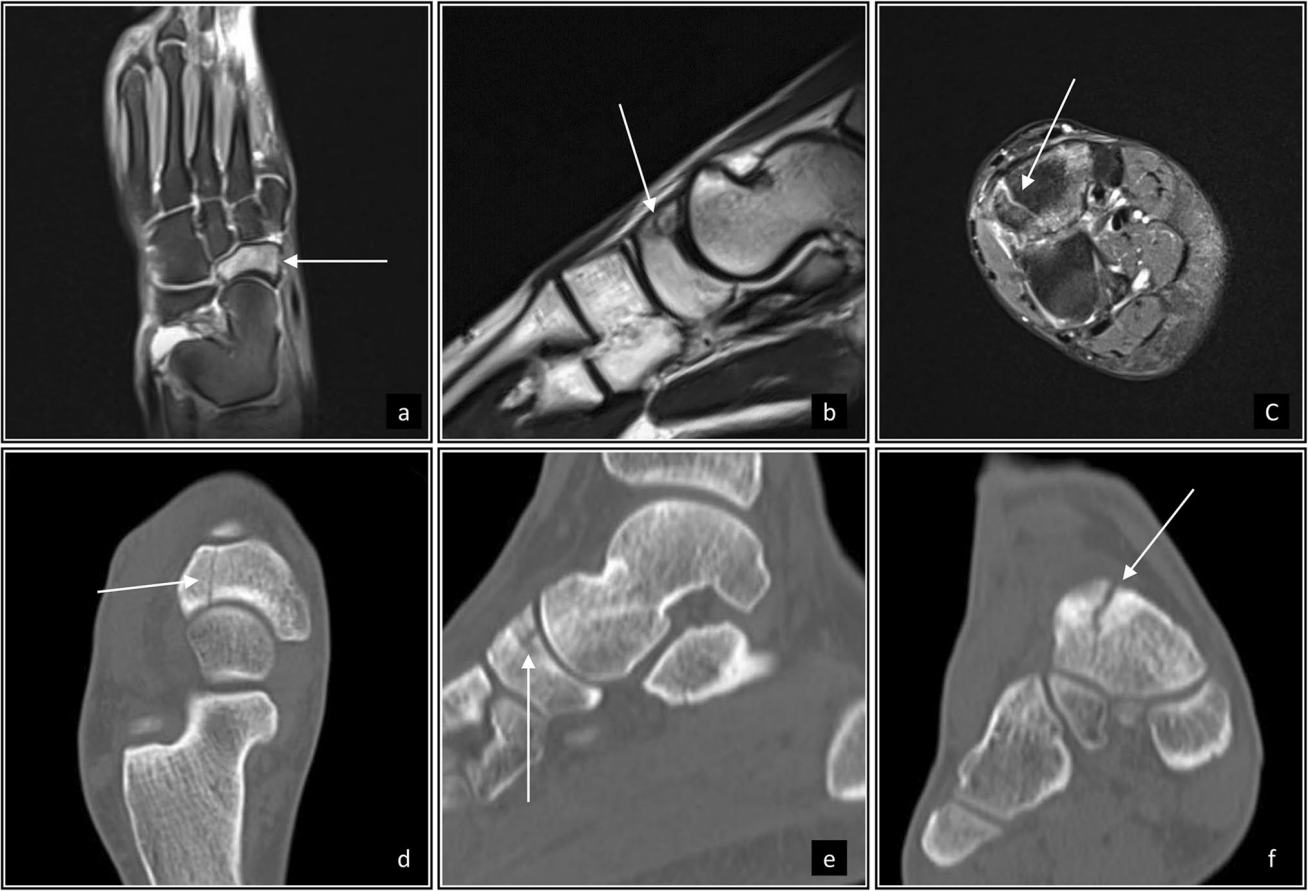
Images from Case 1. Sagittal (a), coronal (b), and long axis (c) on T2 fat-suppressed sequences on magnetic resonance imaging demonstrate vertically oriented stress fracture. Corresponding views on CT visualize the fracture orientation (d–f) and classify as navicular Type III stress fracture [20]
